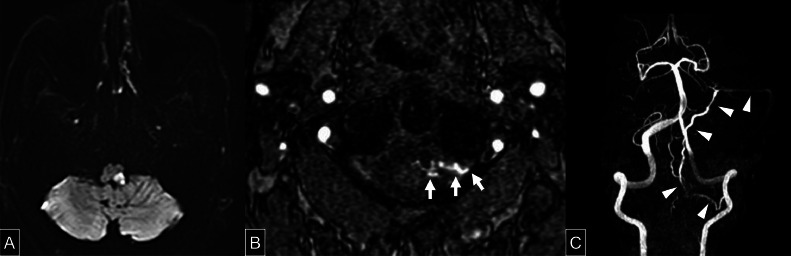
(A) Axial diffusion-weighted MRI showing a lesion with restricted diffusion in the left lateral medulla. (B) Axial MR angiographic image at the level of the second cervical vertebra. A white arrow indicates abnormal vessels extending from the epidural space toward the spinal cord. (C) MR angiographic image with the bilateral internal and external carotid arteries digitally subtracted. A white arrowheads indicates a vessel arising from the left vertebral artery, coursing medially and then ascending to the superior petrosal sinus.
